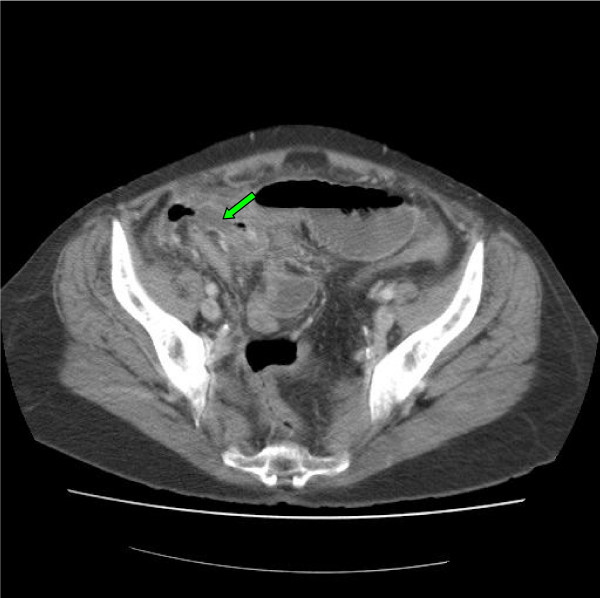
CT of the abdomen of patient 2 showing the inflamed appendix (green arrow).
